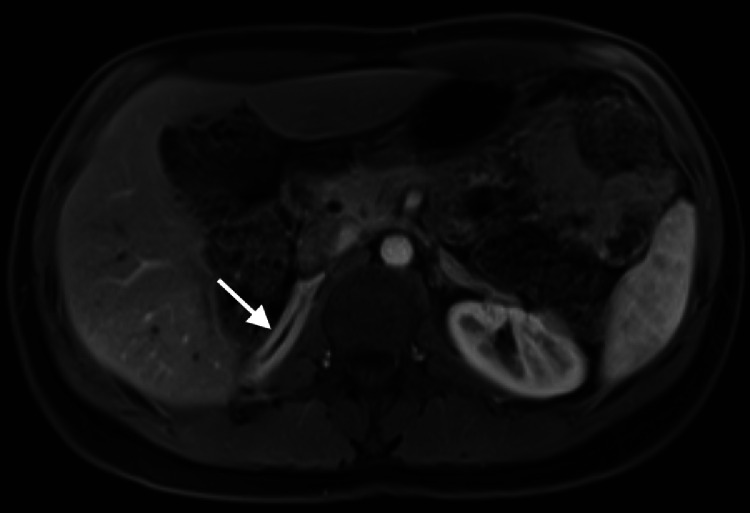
Axial postcontrast-T1 MR image shows homogeneous thickening of the right adrenal gland consistent with adrenal hyperplasia (arrow).
